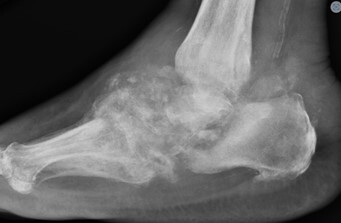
Case 3 lateral foot radiograph showing severe destructive arthropathy with signs of superimposed soft tissue infection and possible osteomyelitis.
